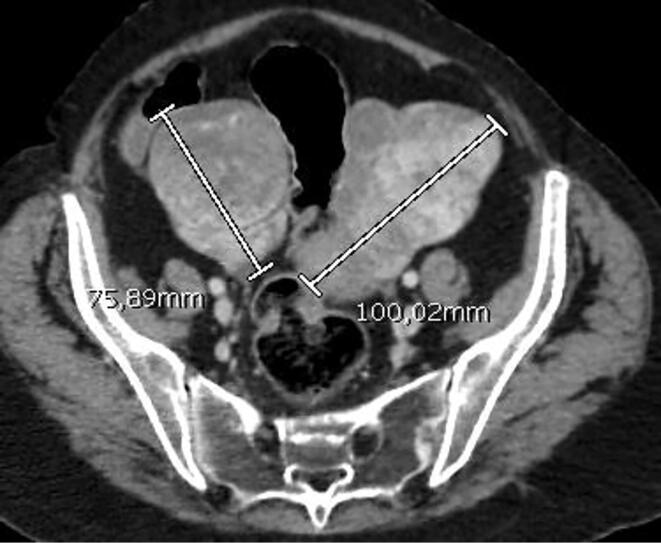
In the axial image of the whole abdominal CT with IV contrast, heterogeneous solid lesions enhanced by contrast with lobulated contours are seen in both adnexal regions.
